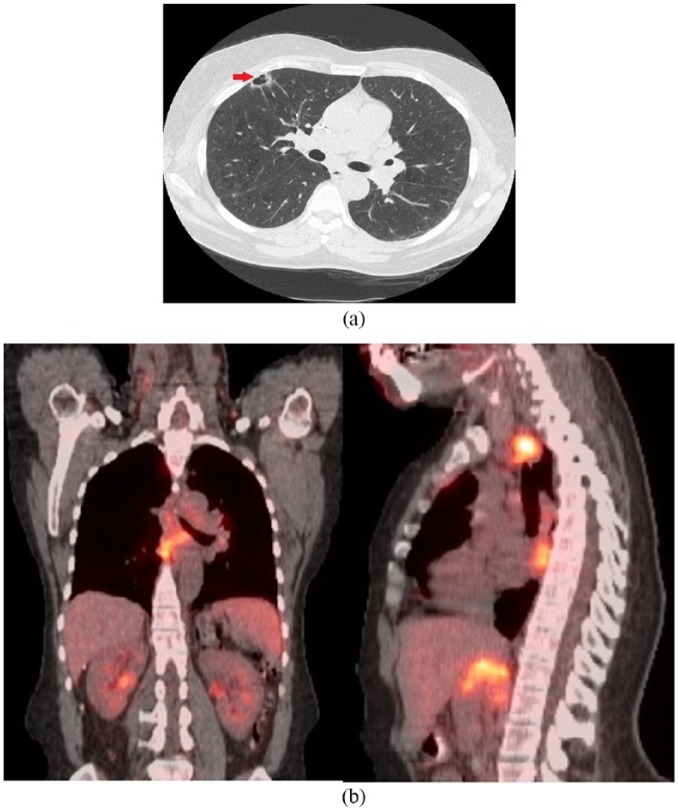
(a) Computed tomography scan of chest showing a 1.7 × 1.1 cm right upper lobe cavitary nodule. (b) Positron-emission tomography scan showing extensive lymphadenopathy both above and below the diaphragm but no uptake in the right upper lobe cavitary lesion.
